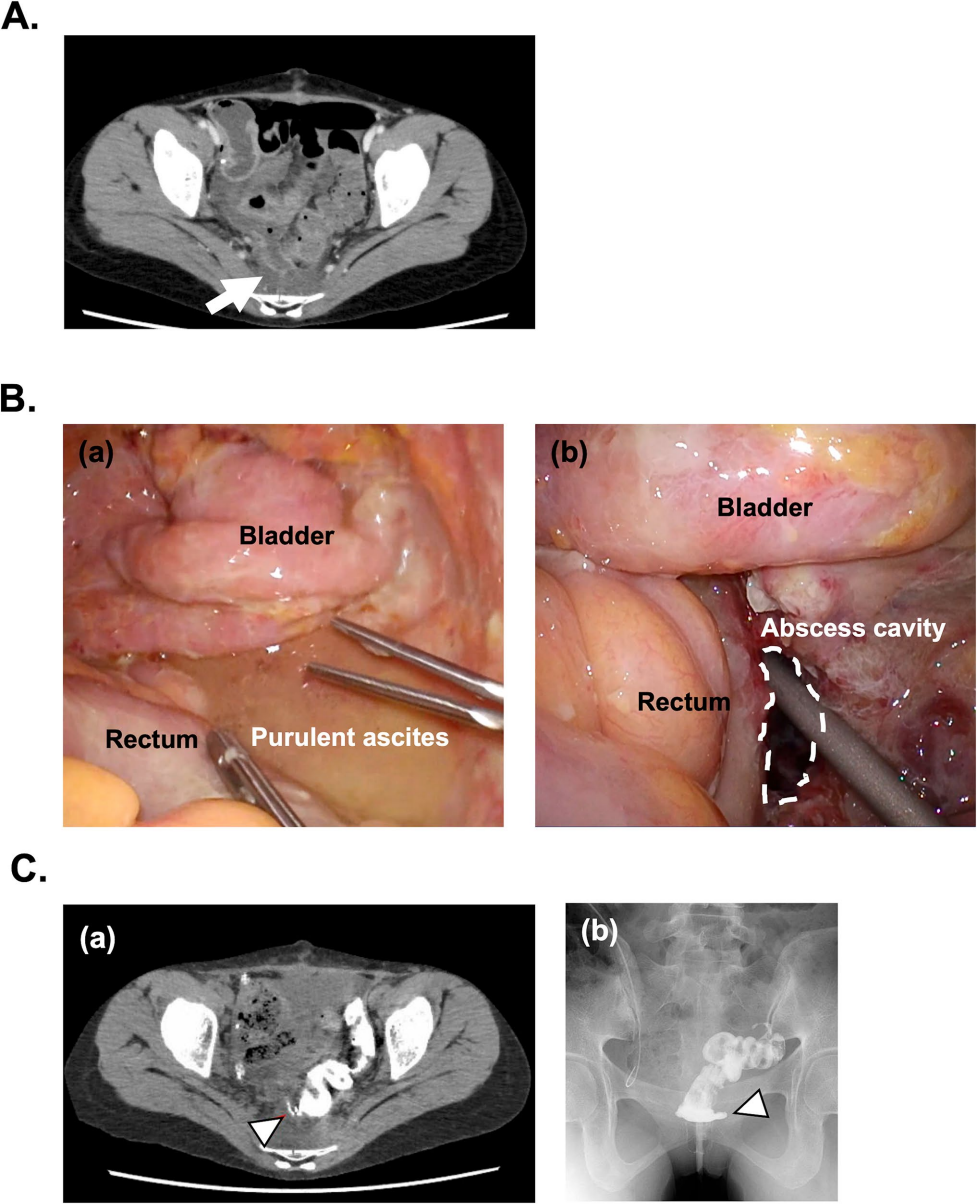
Rectal anastomotic leakage and second operation. A. Computed tomography on postoperative day 11. Intraabdominal free air (arrowhead) and pelvic abscess around rectal anastomosis (arrow) were found. B. Intraperitoneal findings by exploratory laparoscopy. a Purulent ascites was observed around the anastomotic site. b Pelvic abscess was removed and a drain was inserted into the abscess cavity (dotted area). C. Postoperative contrast enema for detecting rectal anastomotic leakage on second-postoperative day 8. a CT scan following gastrografin contrast enema revealed the back side of leakage around rectal anastomosis (arrowhead). b Gastrografin contrast enema also indicated rectal anastomotic leakage (arrowhead)
